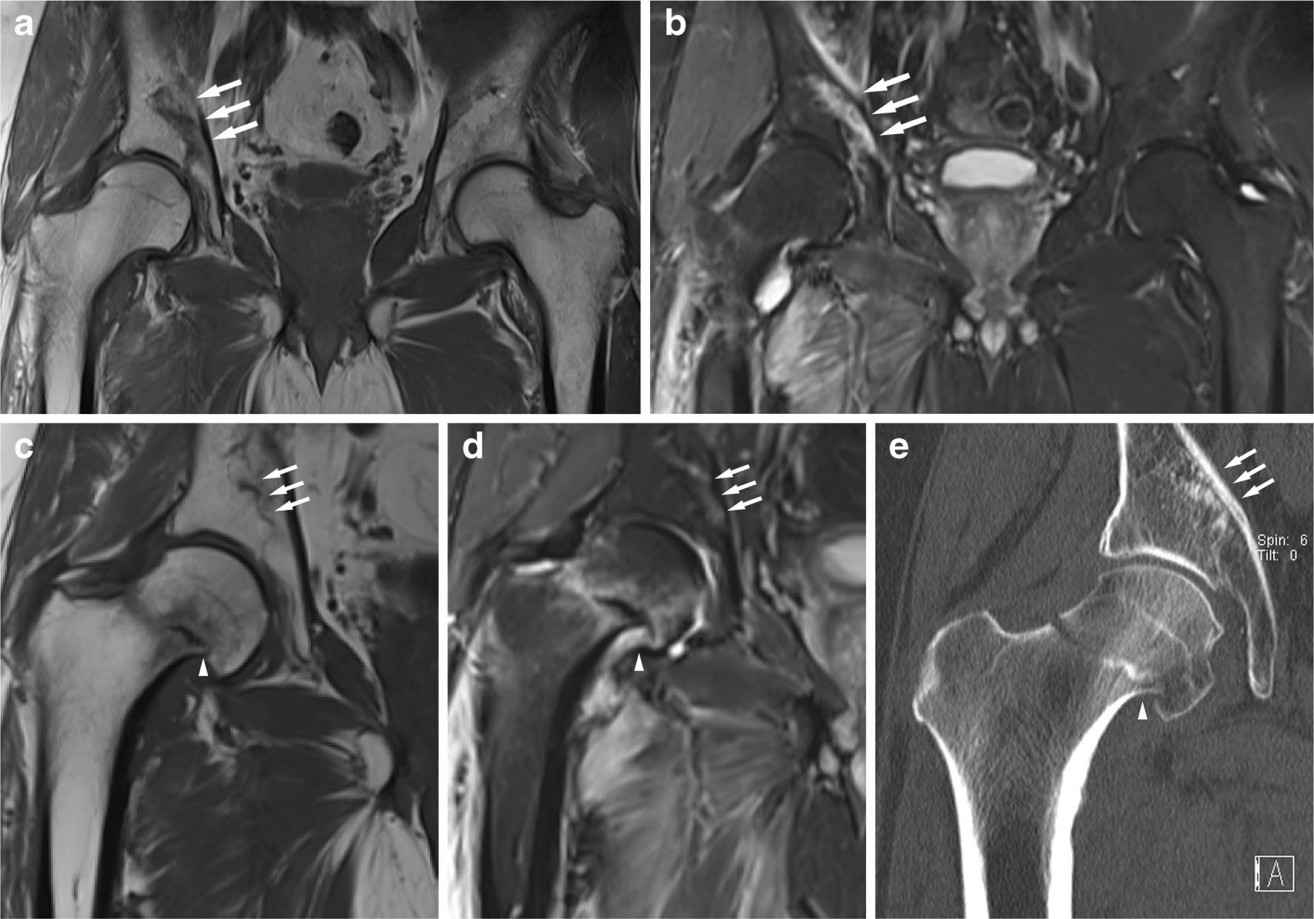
A male patient in his early 60s underwent surgical resection and postsurgical radiotherapy of the right groin and proximal femur for a soft tissue sarcoma. Five months after the radiotherapy the patient experienced right groin pain, and local recurrence was suspected. MRI showed a severe stress fracture of the right acetabulum [coronal T1w (a) and coronal STIR (b) images; see arrows]. Eleven months after the radiotherapy the patient developed a spontaneous right femoral neck fracture after prodromal pain. Coronal MRI (c T1w, d STIR) shows the absence of an underlying malignancy, an impacted femoral neck fracture (arrowhead) and bone marrow with high fat content. The stress fracture of the acetabular roof has largely healed (arrows). There is persistence of marked soft tissue oedema after radiotherapy. CT imaging (e, coronal reformat) also demonstrates the femoral neck fracture (arrowhead) and shows mild sclerosis of the bone marrow in the femoral head and neck, and no obvious osteopenia. The acetabular stress fracture can be appreciated as an area of sclerosis (arrows). Radiotherapy impairs the bone repair capability and predisposes the patient to stress fractures, often in the first year after radiotherapy
